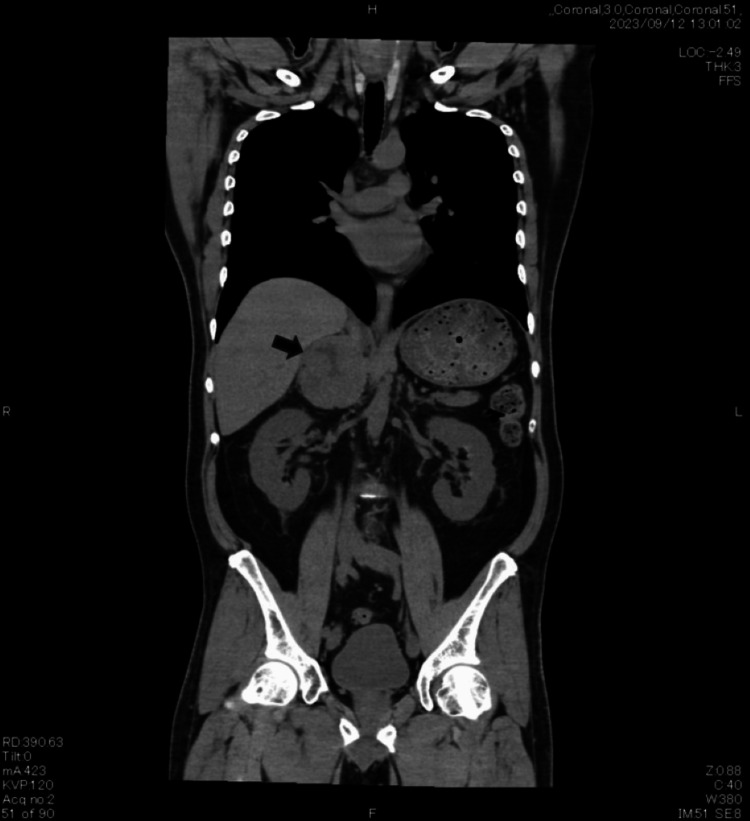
Computed tomography of the right adrenal pheochromocytoma Computed tomography shows a well-defined mass measuring approximately 7 cm in diameter in the right adrenal gland (arrow).
